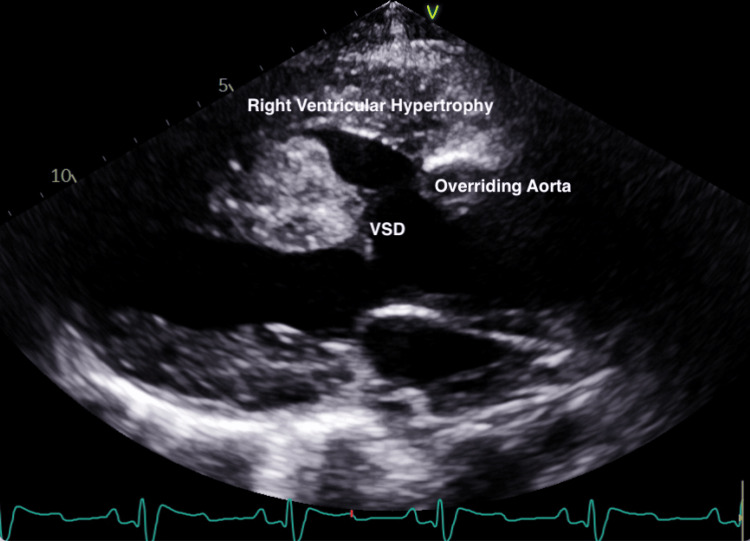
Echocardiogram showing VSD, overriding aorta, and right ventricular hypertrophy
